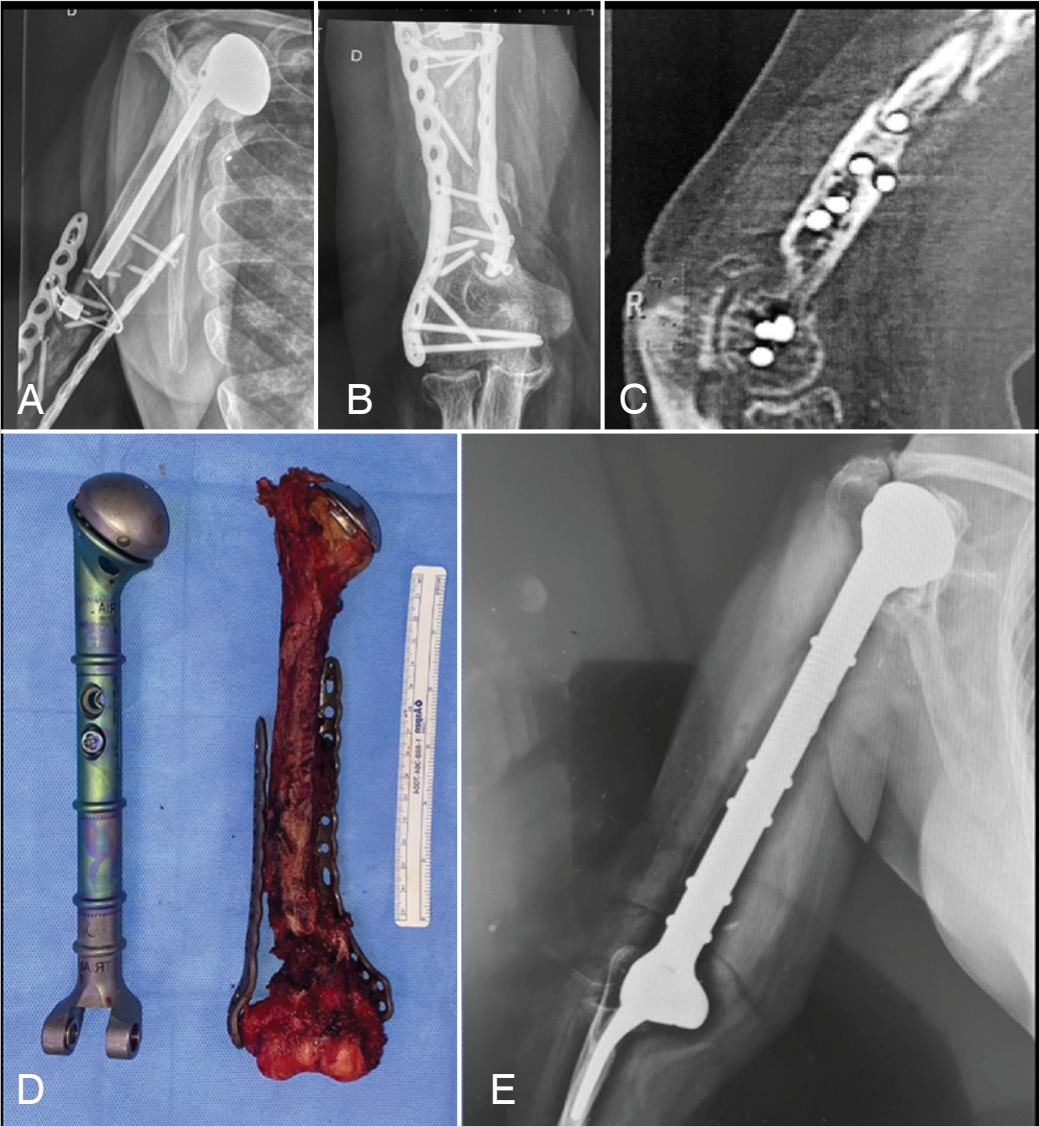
Images of the patient 12. X-rays and tomography showing the two focuses of pseudoarthrosis, fracture and release of the implant and the complete prosthesis of the shoulder (A, B and C). Image of the resected humerus and evidence of the endoprosthesis model used (D). Final X-ray with total replacement of humerus (E).
